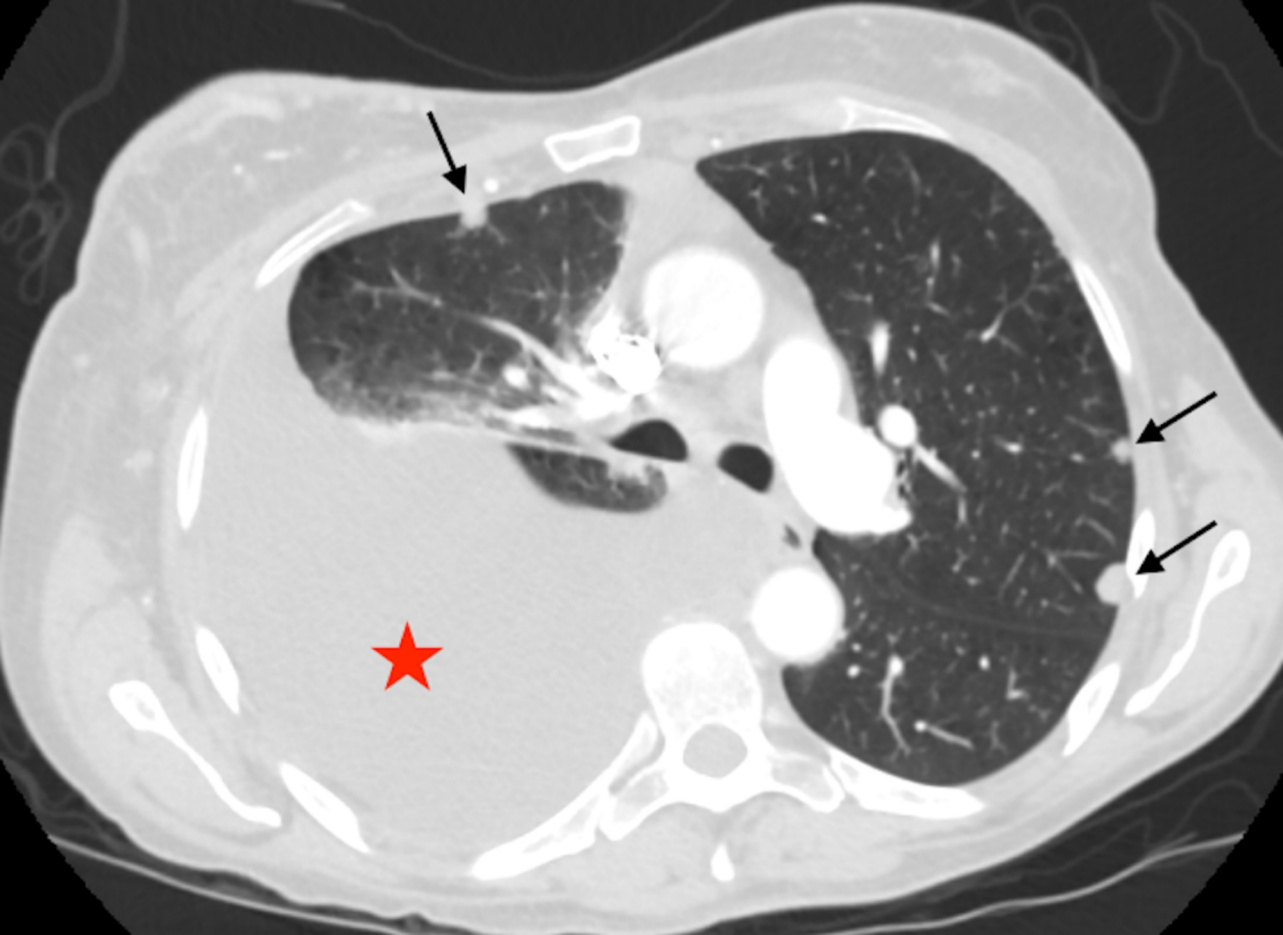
Lung window of an axial image of the CT scan of chest shows bilateral lung nodules (black arrows) and pleural effusion (red star)
